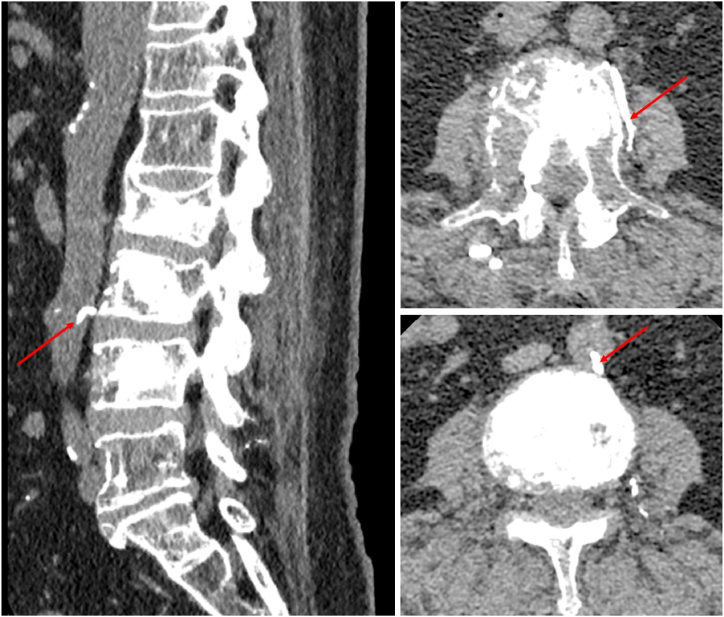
Postoperative lumbar CT sagittal and transverse views showing linear leakage of the third lumbar segmental artery (red arrows).
